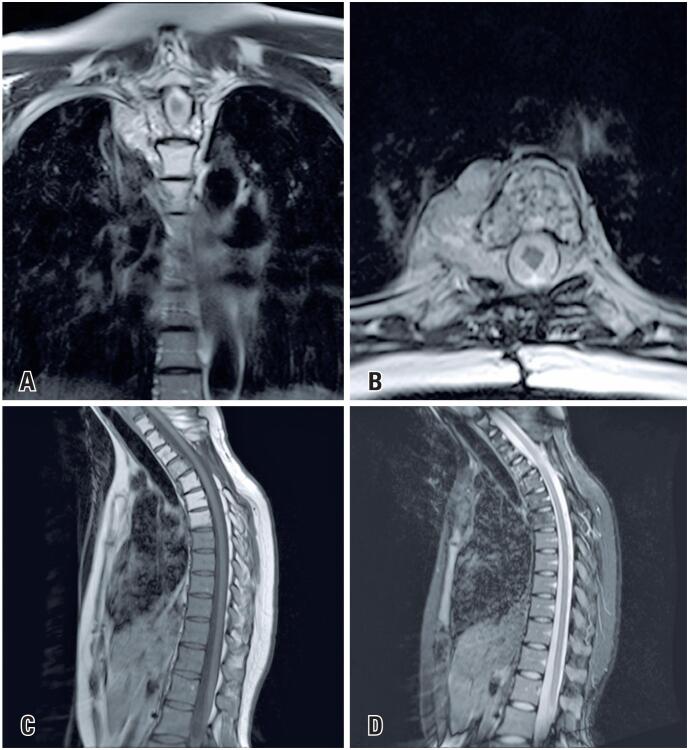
Late magnetic resonance imaging of the thoracic spine. A post-radiotherapy control study with actinic liposubstitution of the vertebral bone marrow and a slight reduction in the solid neoplastic mass at T3 compared with that of the initial examination, and a compressive effect on the spinal cord
